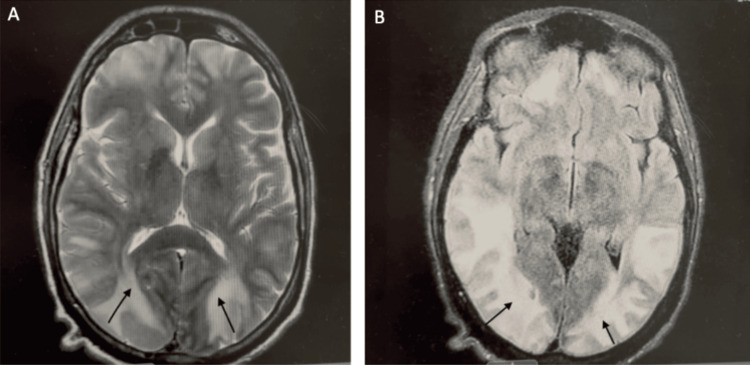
Axial fluid-attenuated inversion recovery (FLAIR) and T2-weighted MRI sequences of the brain showing posterior abnormalities. Axial fluid-attenuated inversion recovery and T2-weighted MRI sequences demonstrate bilateral subcortical and cortical hyperintensities in the parieto-occipital regions (arrows). (A) Axial FLAIR image showing symmetric posterior hyperintensities. (B) Axial T2-weighted image confirming bilateral parieto-occipital involvement.
